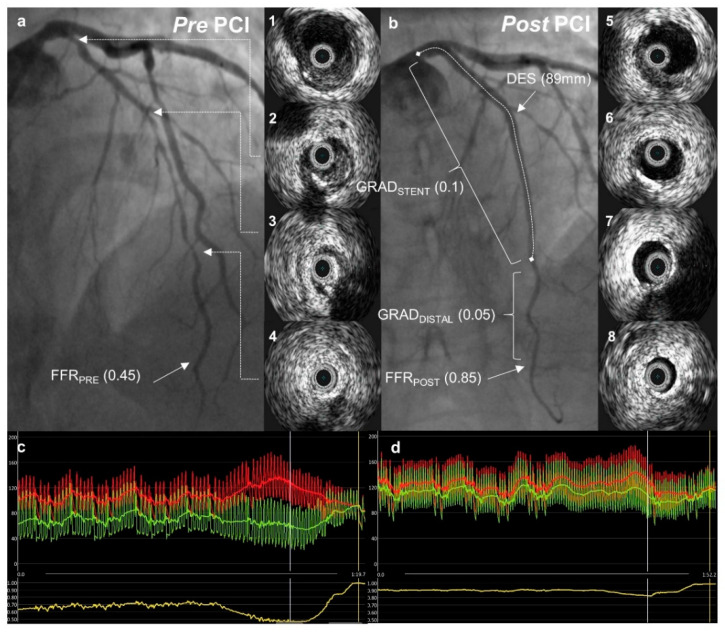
A case example. Pre- and post-percutaneous coronary intervention (PCI) angiographic images with intravascular ultrasound pictures from a corresponding left anterior descending artery segment. Fractional flow reserve curves before and after PCI are presented below. (a) Angiographic image demonstrating diffuse coronary artery disease in the left anterior descending artery (LAD); 1–4—intravascular ultrasound (IVUS) images from corresponding LAD segments showing predominantly soft plaque with mild calcification in mid-LAD (2, 3); (c) distal (Pd, green) and aortic (Pa, red) pressures with fractional flow reserve (FFR) pull-back curve (yellow) demonstrating severe ischemia in the distal LAD; (b) angiographic image after percutaneous coronary intervention (PCI) with an improvement in FFR to 0.85; 5–8—IVUS images from corresponding LAD segments after PCI showing acceptable stent apposition and expansion; (d) post-PCI FFR measurement with a pull-back demonstrating a gradual change in pressure gradient.
